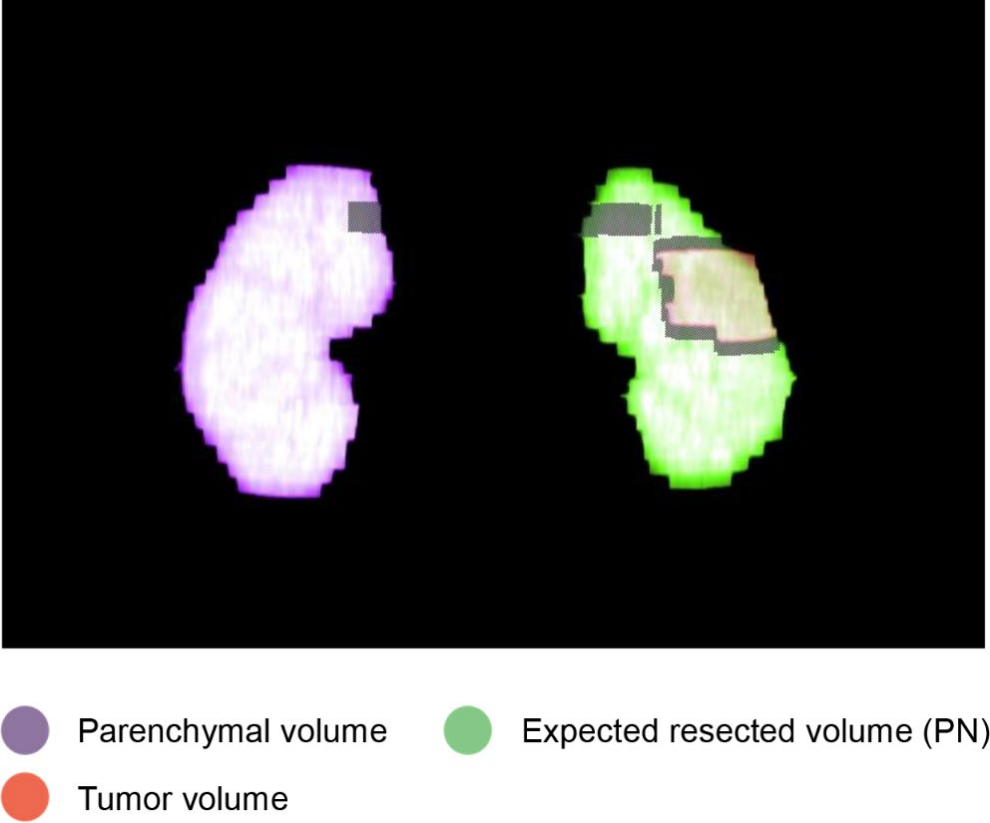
Three - dimensional rendering images of CT volumetric analysis from a radical nephrectomy case. The colors representing different volumes were demonstrated as labeled
